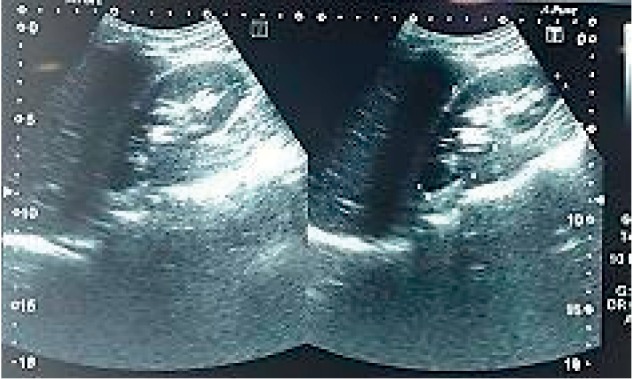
Abdominal ultrasonography showing splenic subcapsular haematoma.
